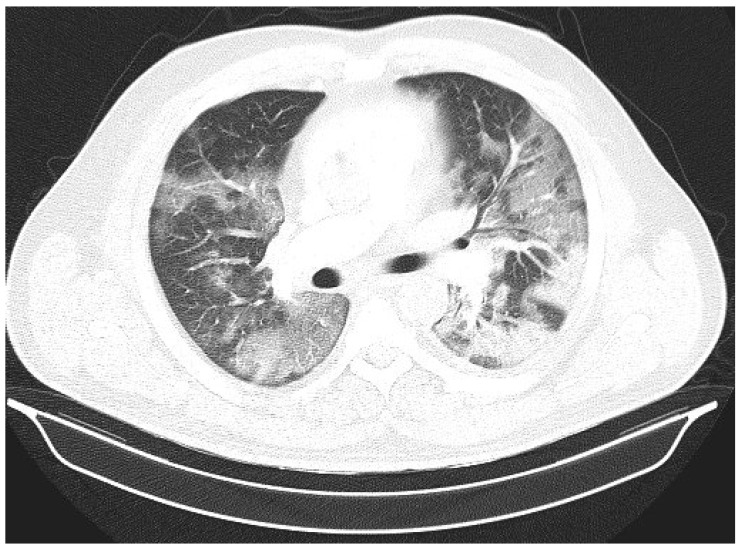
CTPA upon admittance: patient 1. Bilateral ground glass opacifications (severity score: 15–25; chest CT severity score: 14) typical for COVID-19 pneumonia.
